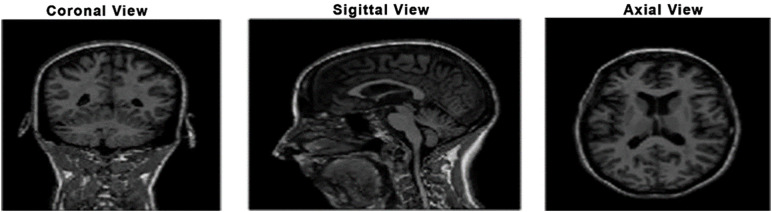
Original 2D MRI views of the brain.
